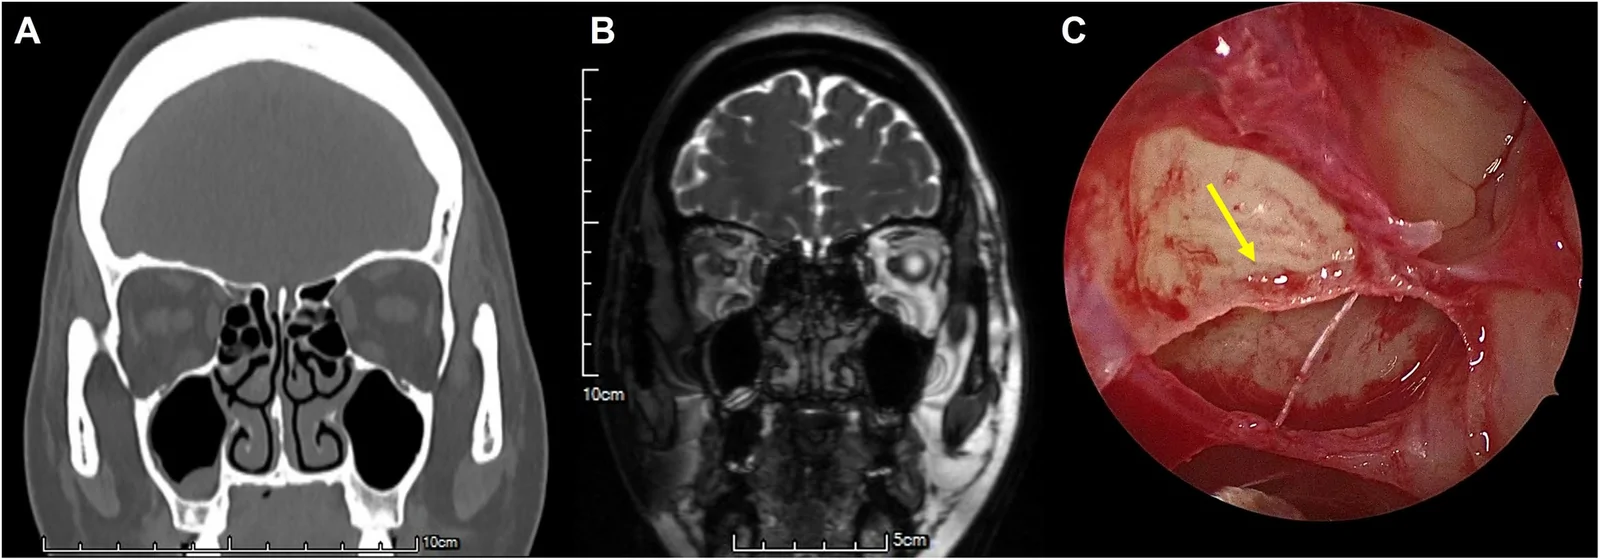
A confirmed ethmoid CSF leak not identified on HRCT (A) or T2W MRI (B) endoscopy (C) located the defect anterior to the anterior ethmoid artery (arrow).
